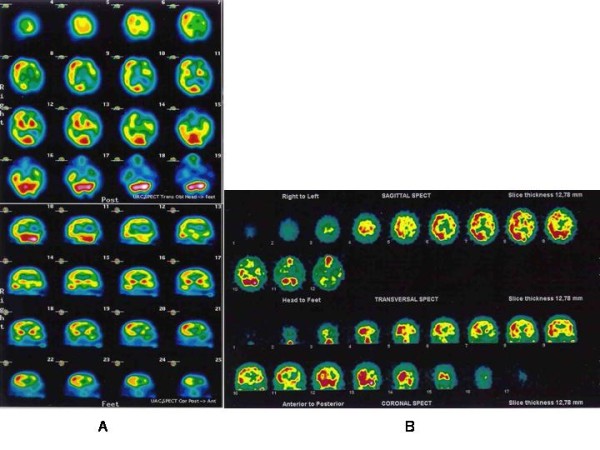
a) SPECT scan eight days later showed decreased perfusion on the posterior portion of the left parietal lobe and inferior temporal lobe area; b) Brain SPECT seven months later revealed severe hypoperfusion in the left hemisphere.
